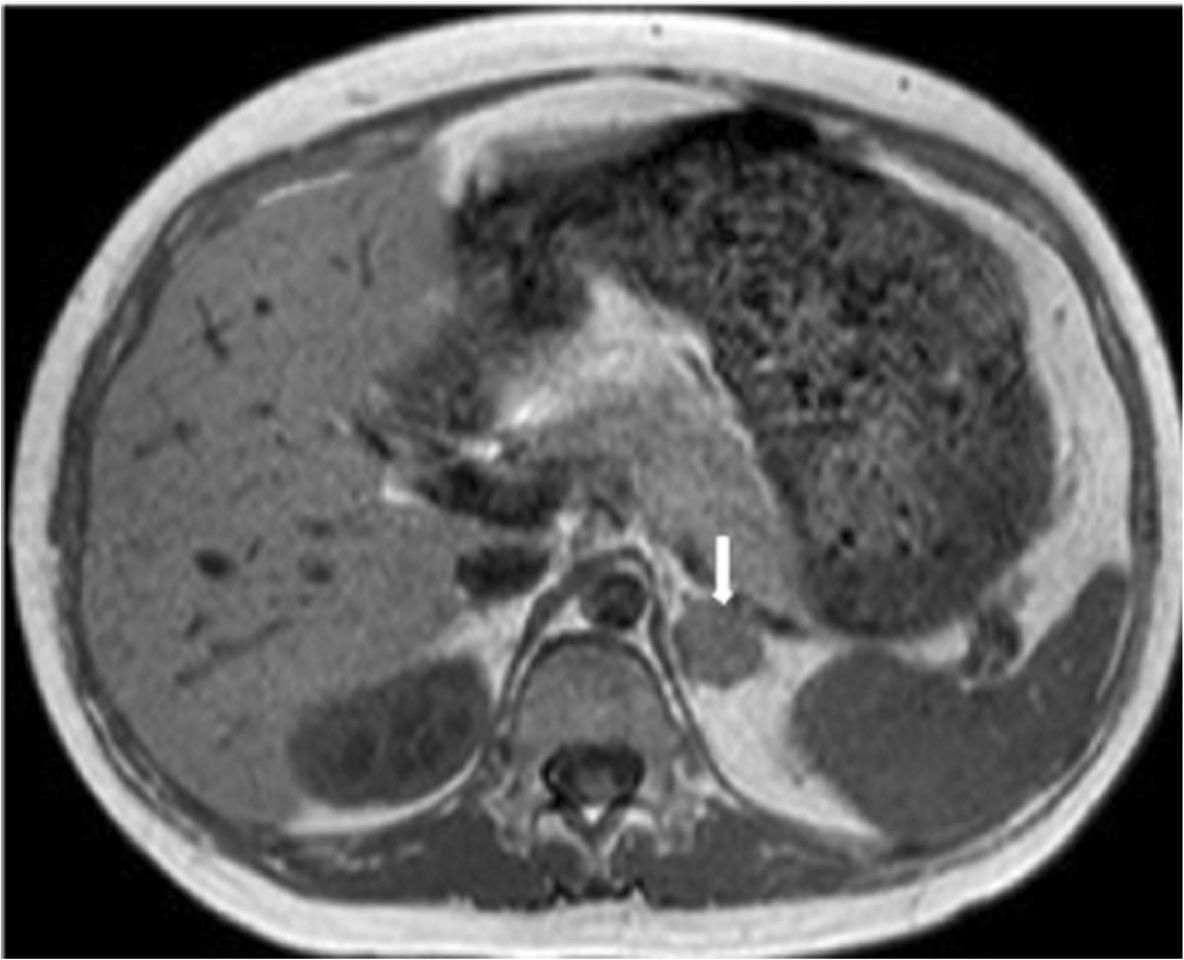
Adrenal magnetic resonance image (MRI) without contrast of the patient. Adrenal MRI showed a left adrenal nodule measuring 2.0 cm in diameter (arrow)
